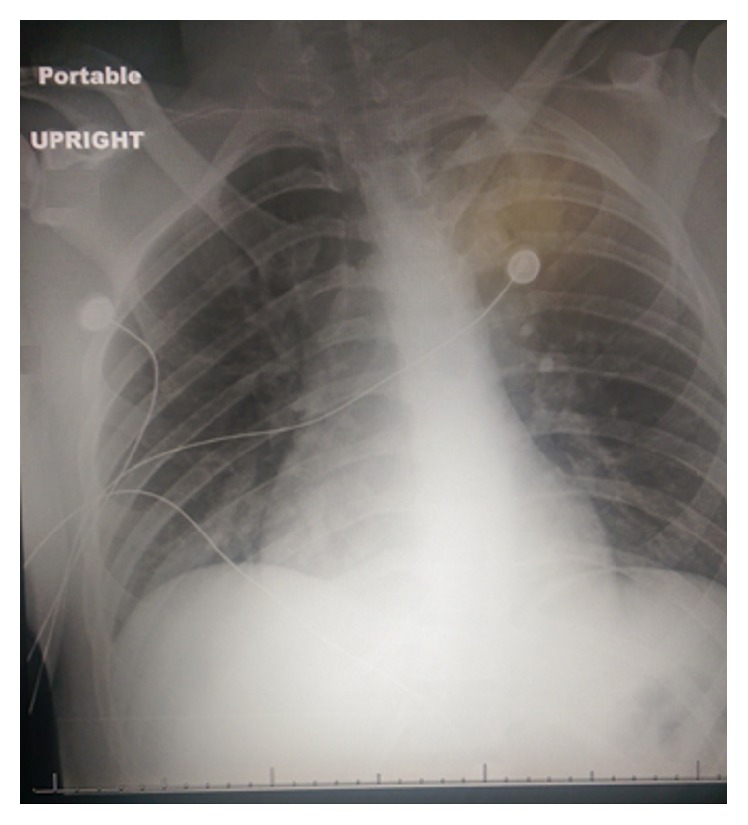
Anterior-posterior chest radiograph showing no obvious abnormalities in the thoracic cavity.
